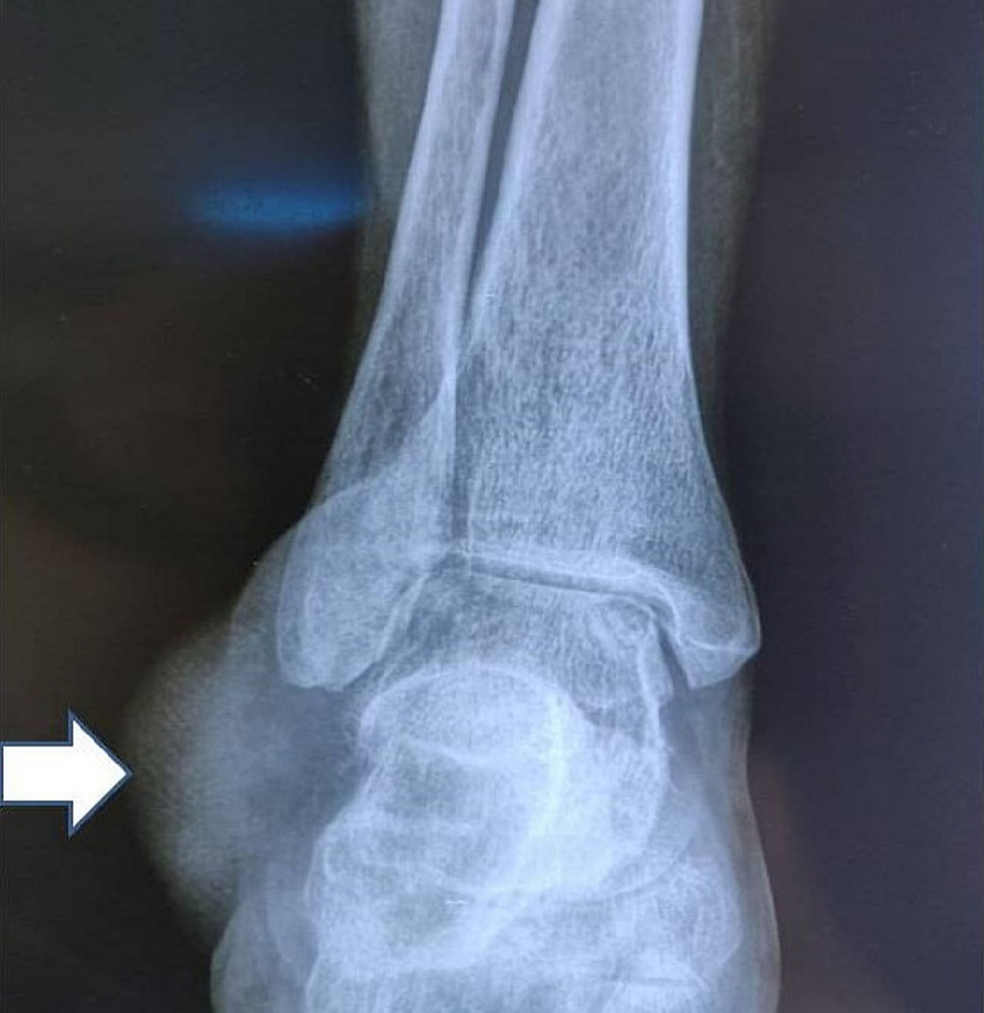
Radiograph showing soft tissue swelling with lucency in the calcaneum
